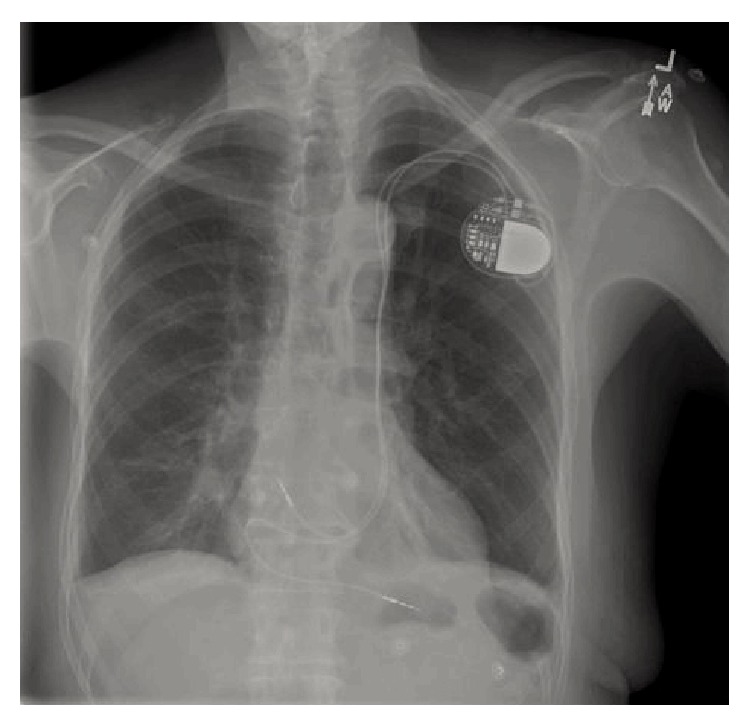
Chest X-ray showing postprocedure implantation of a dual chamber pacemaker, with leads traversing through a persistent left superior vena cava.
